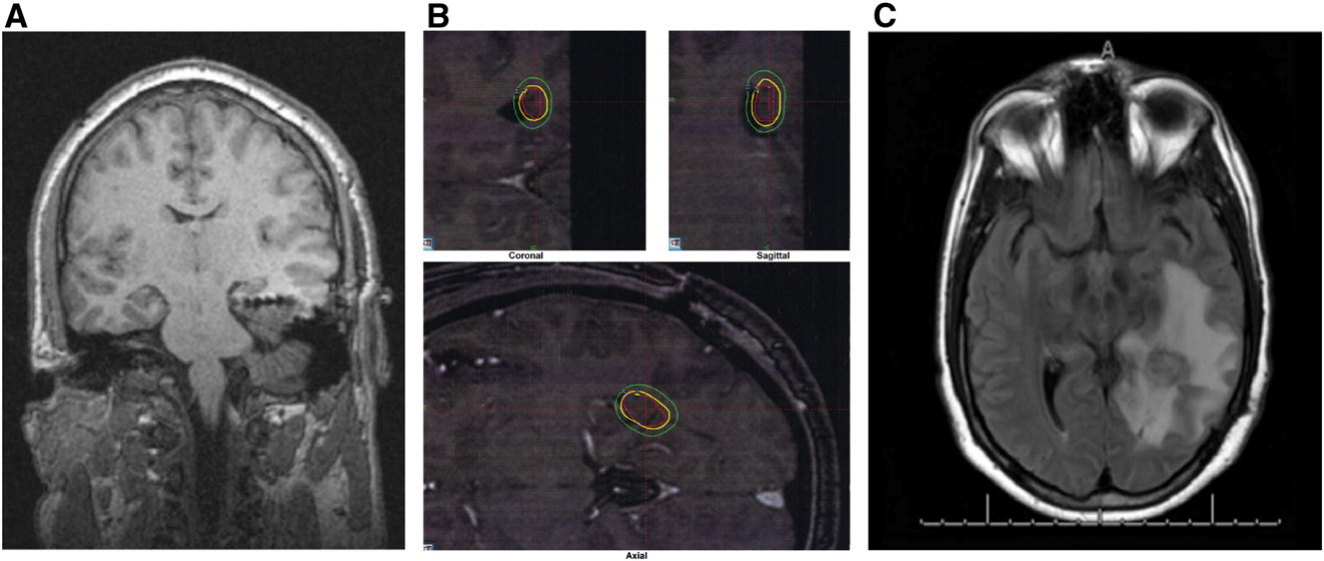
A: Post-operative coronal T1 MRI showing depth electrodes. B: Dose plan for Case 3. C: Axial FLAIR MRI at 19 months post-treatment showing radiation necrosis.
